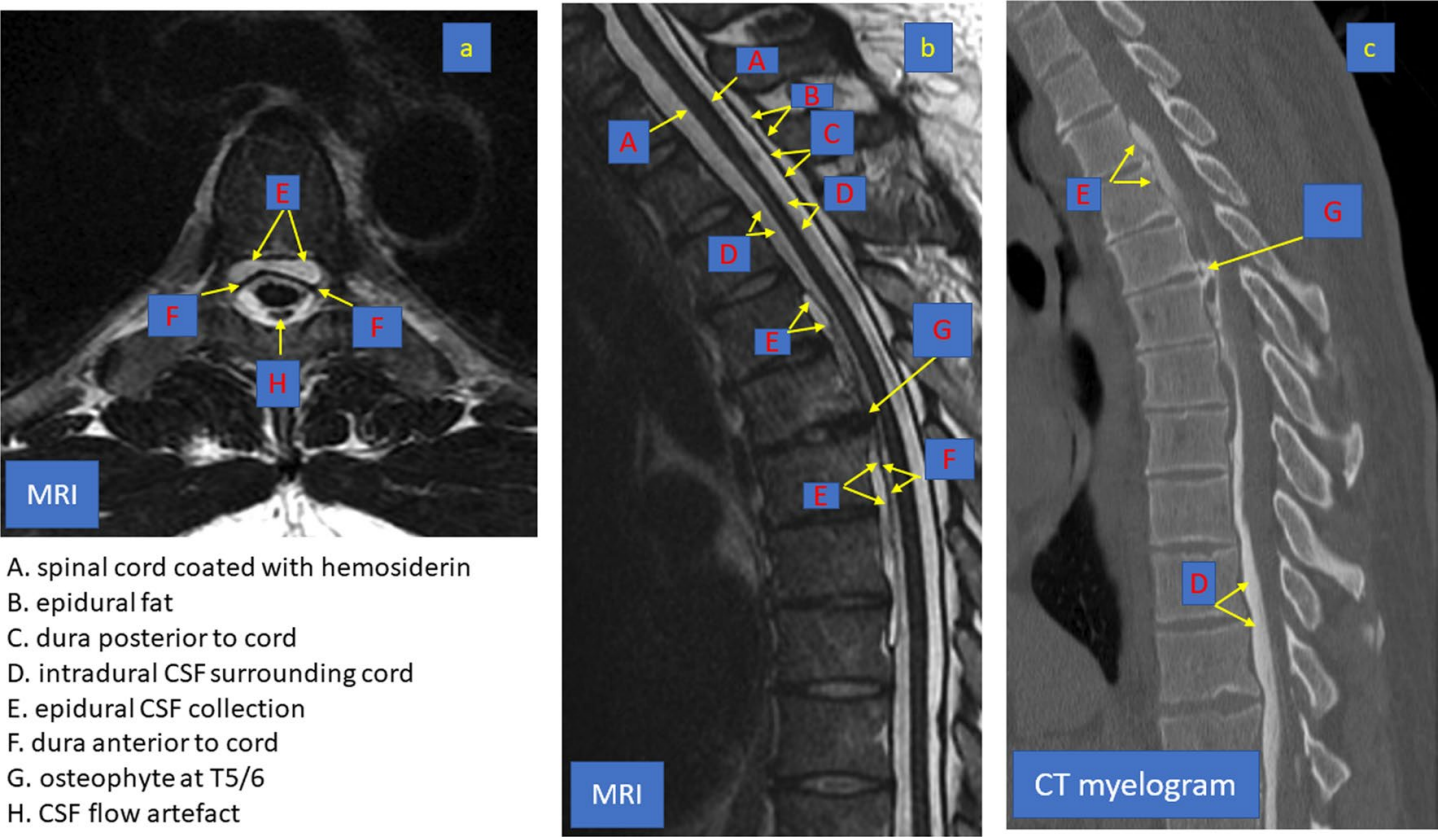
Patient (4). T2 weighted spine MRI in axial (a) and sagittal (b) section showing a longitudinally extensive epidural CSF collection from T2 to T 8 (E) with a large spike osteophyte at T5/T6 impinging on the dura (G). The CT myelogram (c) shows contrast in the epidural collection from a CSF leak adjacent to the osteophyte (G). (A) spinal cord coated with hemosiderin. (B) epidural fat—bright on T2. (C) dura posterior to cord; (D) intradural CSF surrounding cord; (E) epidural CSF collection; (F) dura anterior to cord; (G) osteophyte; (H) CSF pulsation artefact
